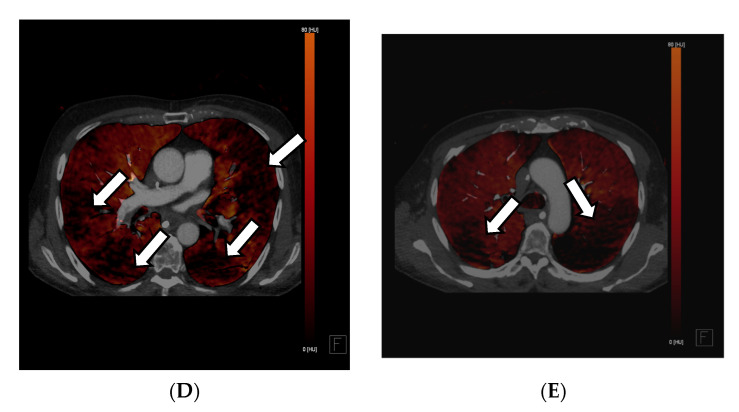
Application of iodine mappings and perfusion visualization facilitating DECT in a DSCT scan of a 23-year-old woman suffering from chest pain, tachycardia, and dyspnea. The 50 kV axial soft tissue kernel reconstruction CT angiography of the pulmonary arteries showed central thrombosis of both main pulmonary arteries on the right side (A,B) and the left side (C) with partial occlusion of the subsequent vessels. V-shaped perfusion deficits were reported in the perfusion maps (D,E), which can be correlated to segments 9/10. In correspondence to the missing contrast agent in the responding segmental arteries of these segments, a total occlusion was suspected. The patient was accordingly treated with systemic anticoagulation.
